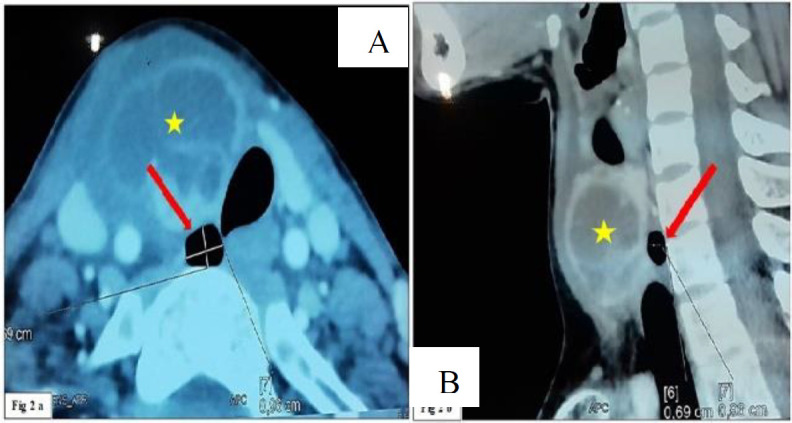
a: Cervical CT (computed tomography) scan in axial section with contrast enhancement shows a ring enhancing cystic lesion located in the right lobe of the thyroid gland ( ) which exerts a mass effect on the trachea, and a 9,6 x 6,9 mm air image ( ) located in the right posterolateral tracheal wall without communication with the lumen of the trachea.. 2b: Cervical CT (computed tomography) scan in sagittal section with contrast enhancement shows a ring enhancing pretracheal cystic lesion ( ) and a 9,6 x 6,9 mm retrotracheal air image ( ).
